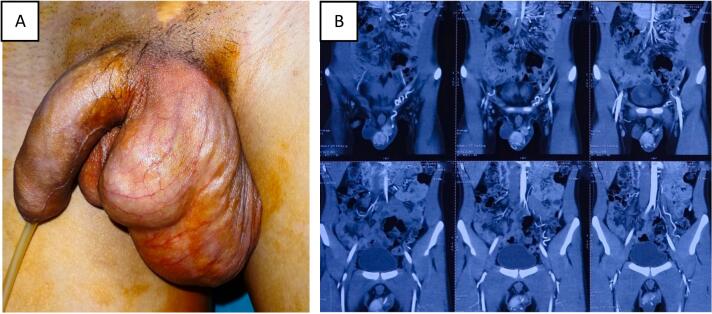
Clinical and subclinical signs. Fig. 1 A. The mass in the left scrotum is continuous with the testicle, and it pushes the testicle downward. Fig. 1 B. Multi-scan computed tomography showed a left scrotal mass consecutive to the lower pole of the native spleen due to SGF disease.
